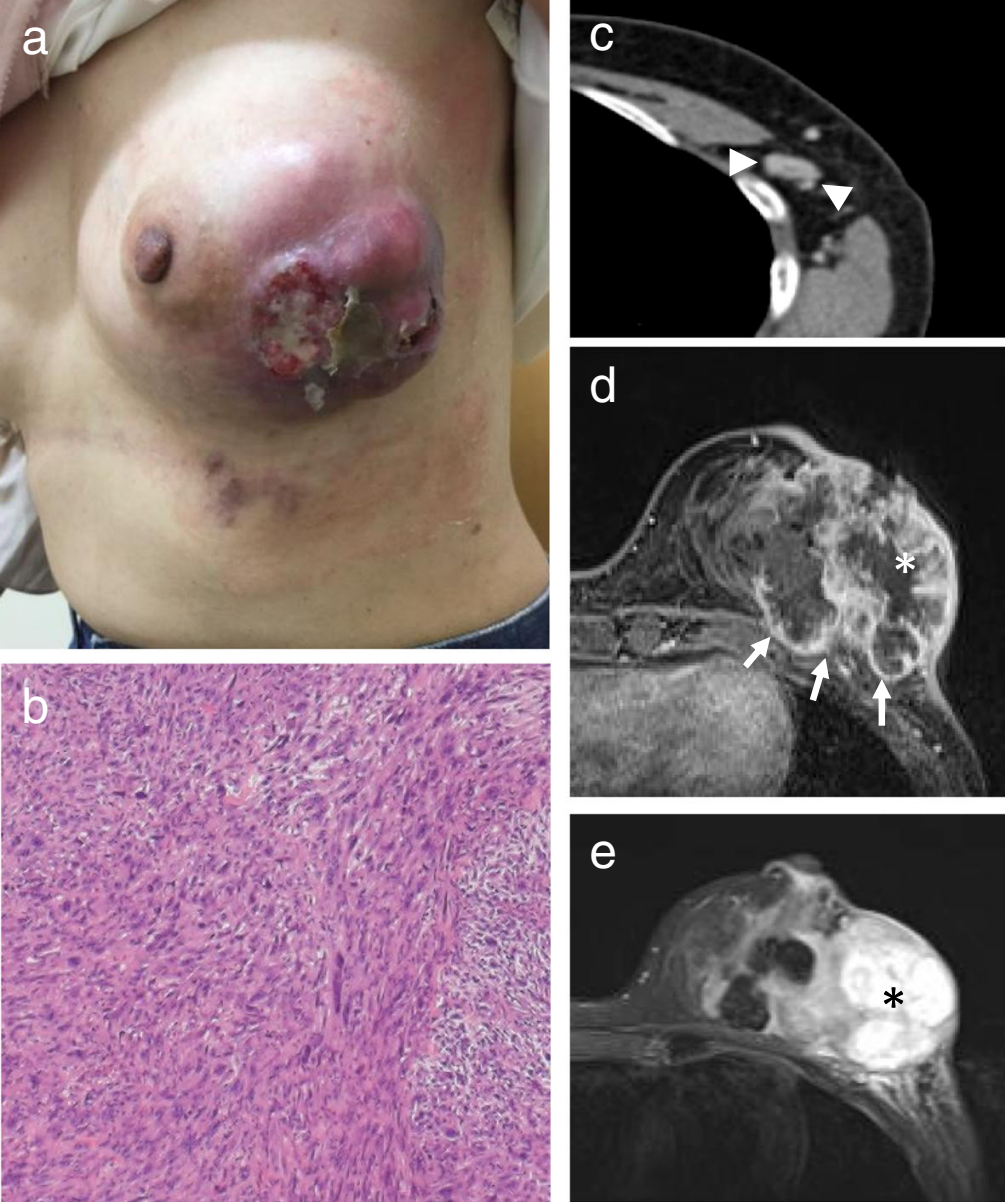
a The bulky mass with ulceration prior to neoadjuvant chemotherapy. b Spindle cells with moderate-to-marked cytologic atypia and mitotic activity (high-power view). c Computed tomography shows the enlarged axillary lymph nodes (arrow heads). d, e T1-weighted fat-saturated contrast-enhanced magnetic resonance imaging shows a 78 × 58 mm tumor adjacent to the pectoralis major muscle with enhancement (arrow) (d). The center of the tumor is necrotic (*asterisk*) based on the low intensity on contrast-enhanced T1-weighted images (d) and high intensity on T2-weighted image (e). The pre-existing necrotic area is considered “pre-treatment necrosis” to differentiate it from post-treatment necrosis caused by neoadjuvant chemotherapy
